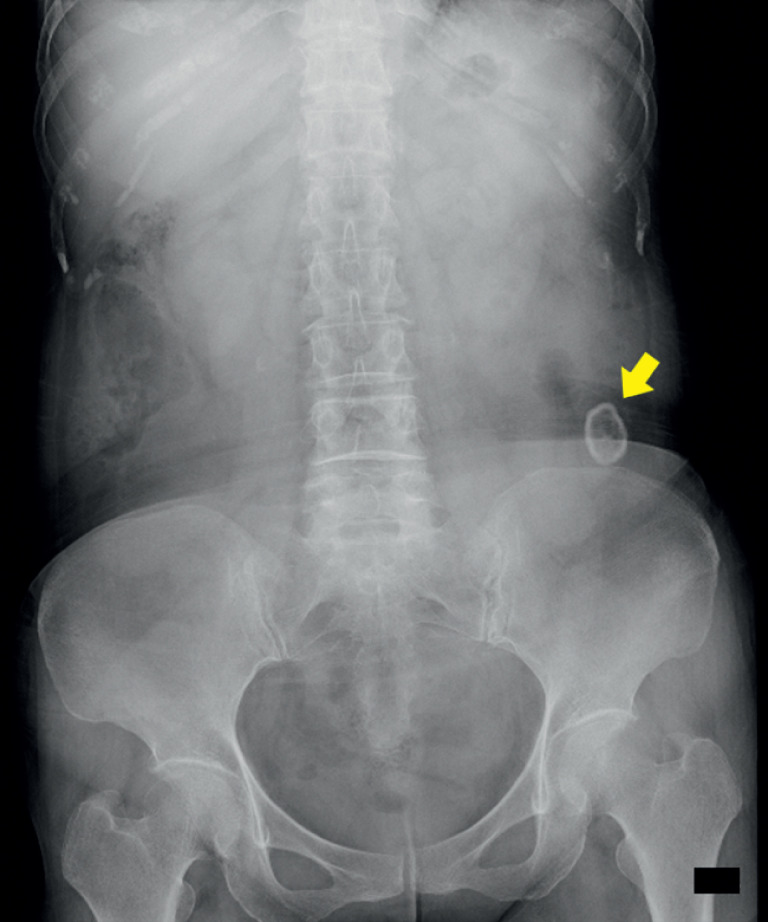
Abdominal X-ray showing a 2.6-cm stone located in the left lower part of the abdomen (yellow arrow).
